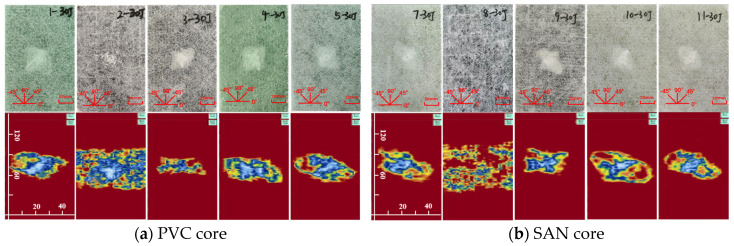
Physical picture of 30 J impact damage and ultrasonic C-scan image.
